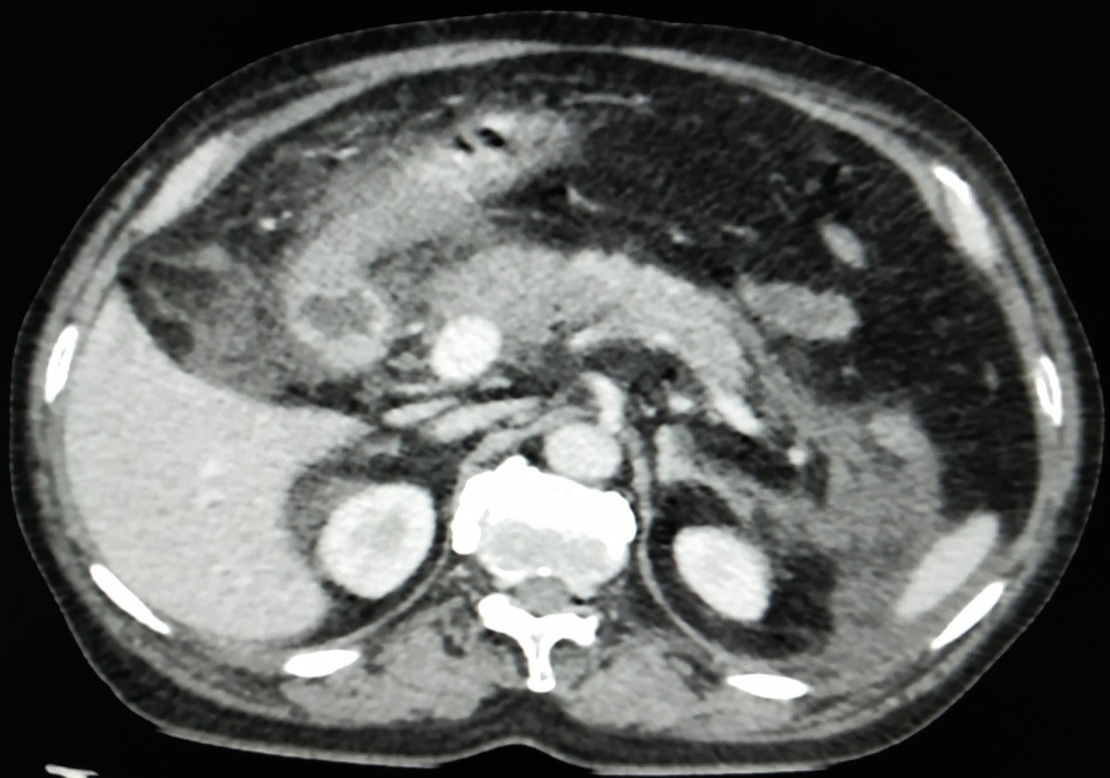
Contrast enhanced computed tomography of abdomen shows enlarged tail, distal part of body with indistinct margin, normal enhancement and peritoneal collection.
